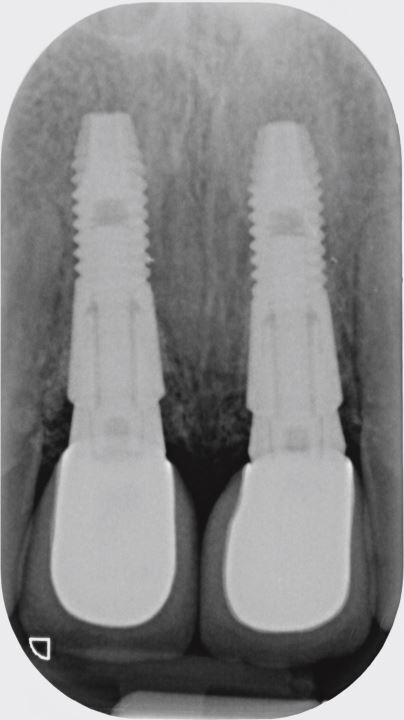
Radiograph showing apparent good radiographic bone levels
